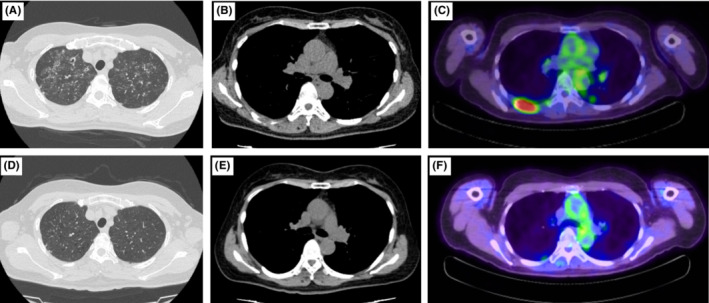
Chest computed tomography (CT) of the (A) lung and (B) bone lesions on Day 1. (C) FDG‐PET/CT on Day 11. CT of the (D) lung and (E) bone lesions as well as (F) FDG‐PET/CT approximately 2 years after the initial visit.
